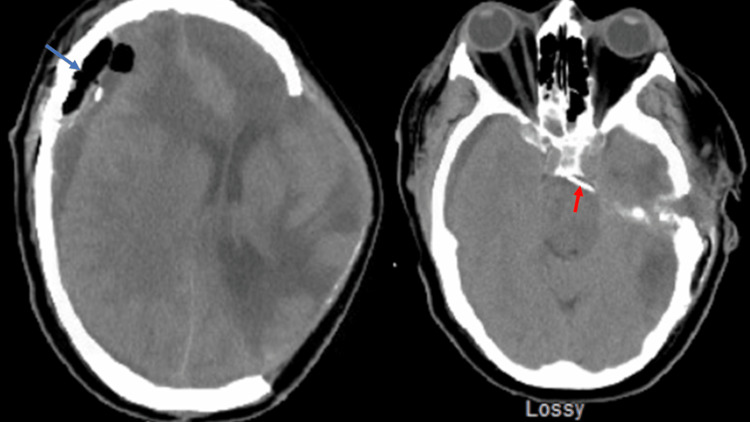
Postoperative axial CT. The view shows extra-axial fluid thought to be CSF (blue arrow) and the inserted catheter in the prepontine cistern (red arrow).
